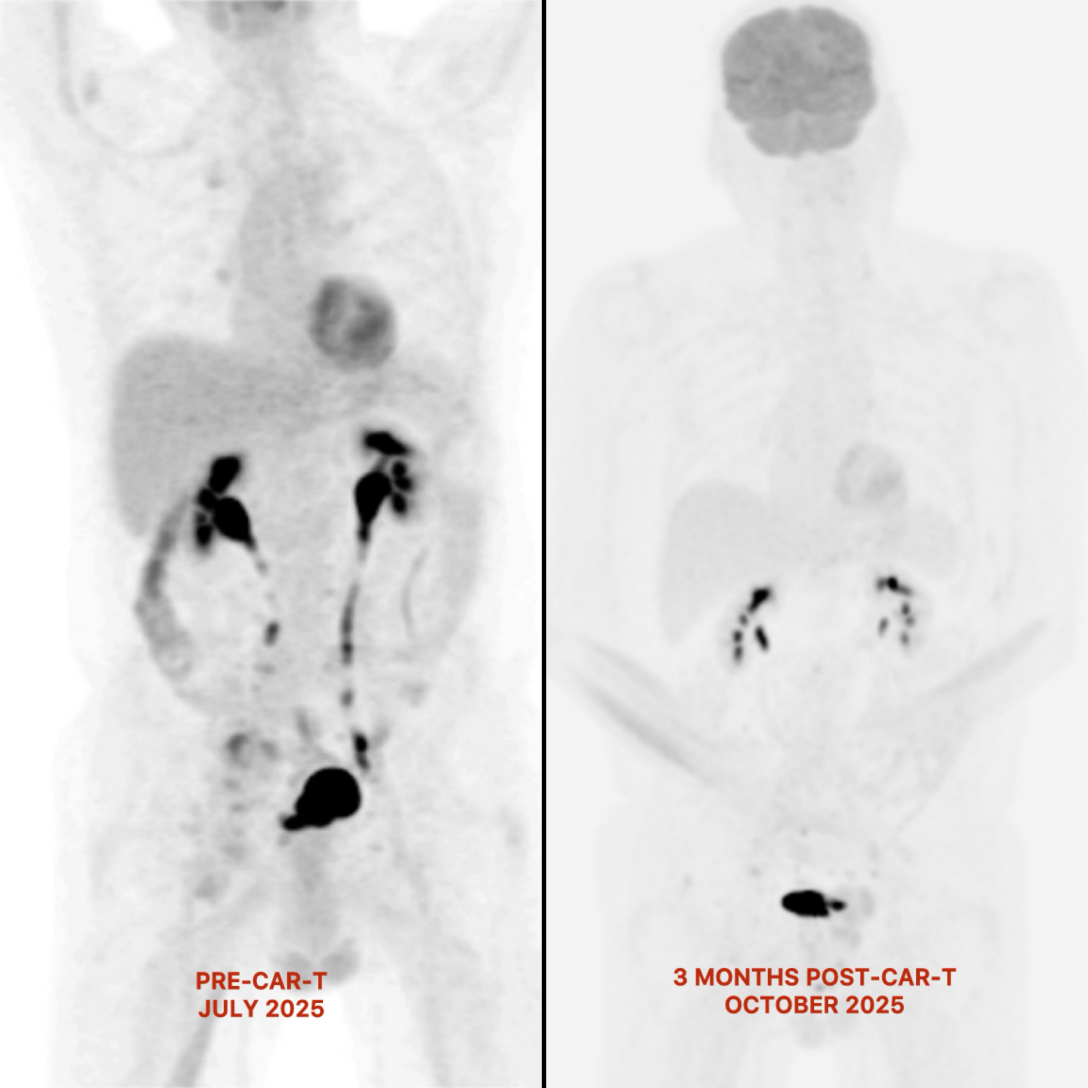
Changes in metabolic activity pre- and post-CAR-T therapy on PET-CT scan. No residual hypermetabolic nodular disease in the retroperitoneum or mesentery, with a marked bulk reduction in the right inguinal and common iliac lymphadenopathy 3 months after CAR-T therapy.
